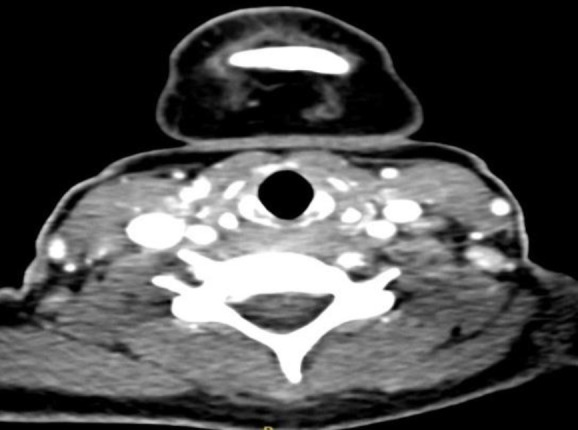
Contrast enhanced CT scan shows multiple lymph nodes with heterogenous enhancement on both sides of the neck with no necrotic component and some of them are matted.
